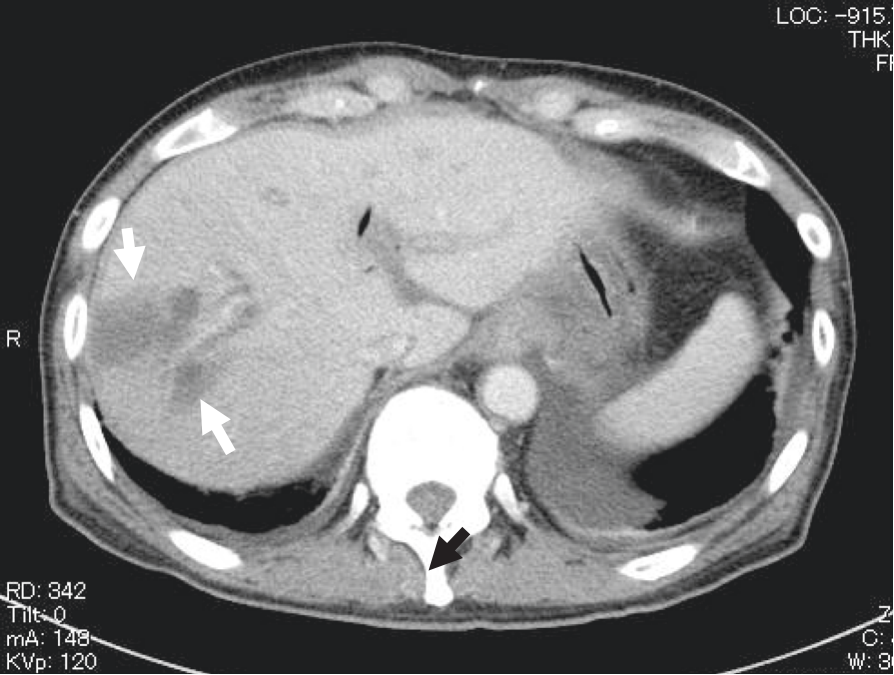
CT image which has been performed 14 days after stent graft placement. A low-density area corresponding to the liver abscess was observed in the right hepatic lobe
